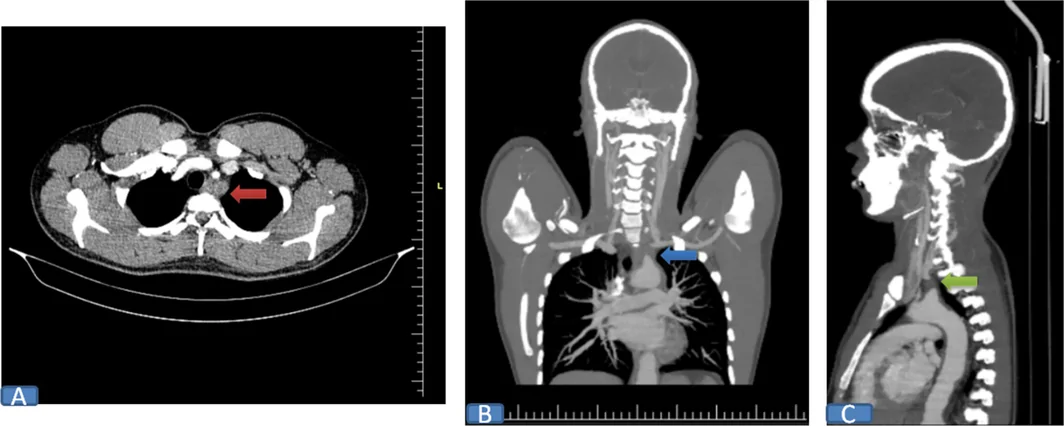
CT angiography demonstrates complete proximal left subclavian artery occlusion, seen as abrupt contrast cutoff on axial image (A, red arrow), coronal image (B, blue arrow), and sagittal image (C, green arrow).
